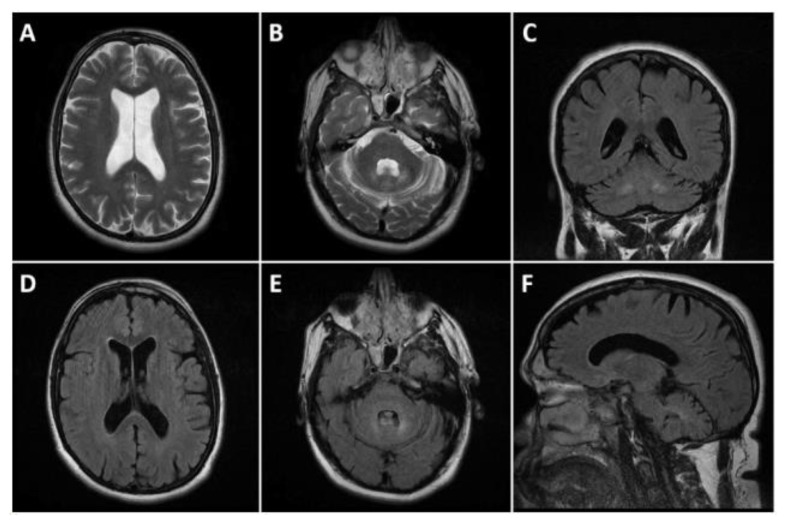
Head MRI presenting cortical atrophy and bilateral changes in the signal intensity in the zones of the dentate nucleus. (A,B): T2 axials; (D,E): FLAIR axials; (C): FLAIR coronal, and (F): FLAIR sagittal. Dilated ventricular system and subarachnoid fluid spaces on the convexity bilaterally (A,D). Symmetric T2 and FLAIR hyperintensities affecting the dentate nuclei (B,C,E,F).
